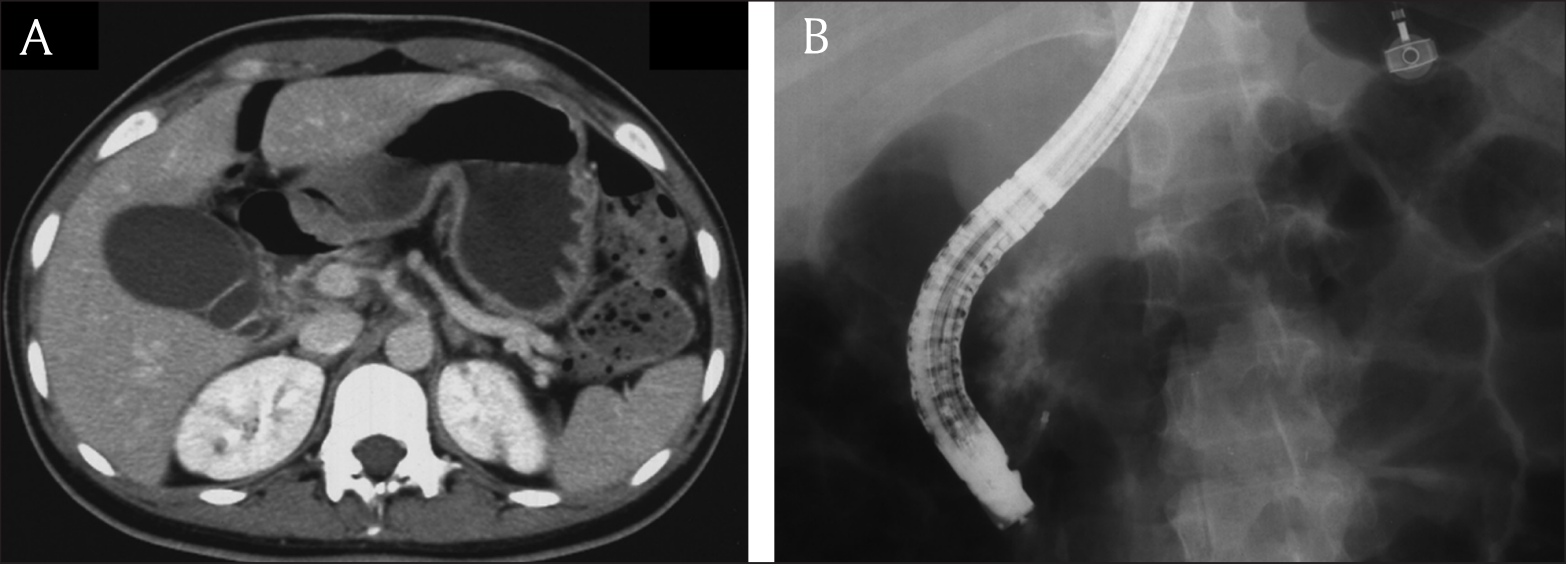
A 22-year-old woman with dorsal pancreas agenesis. (A) Axial portal venous phase CT image shows absence of dorsal pancreas. Note stomach in the distal pancreatic bed. (B) Endoscopic retrograde cholangiopancreatography image shows absence of dorsal pancreatic duct.
